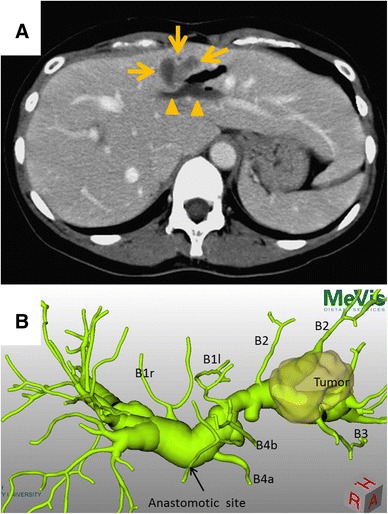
Enhanced abdominal computed tomography and three dimensional-drip infusion cholangiography-computed tomography. a
